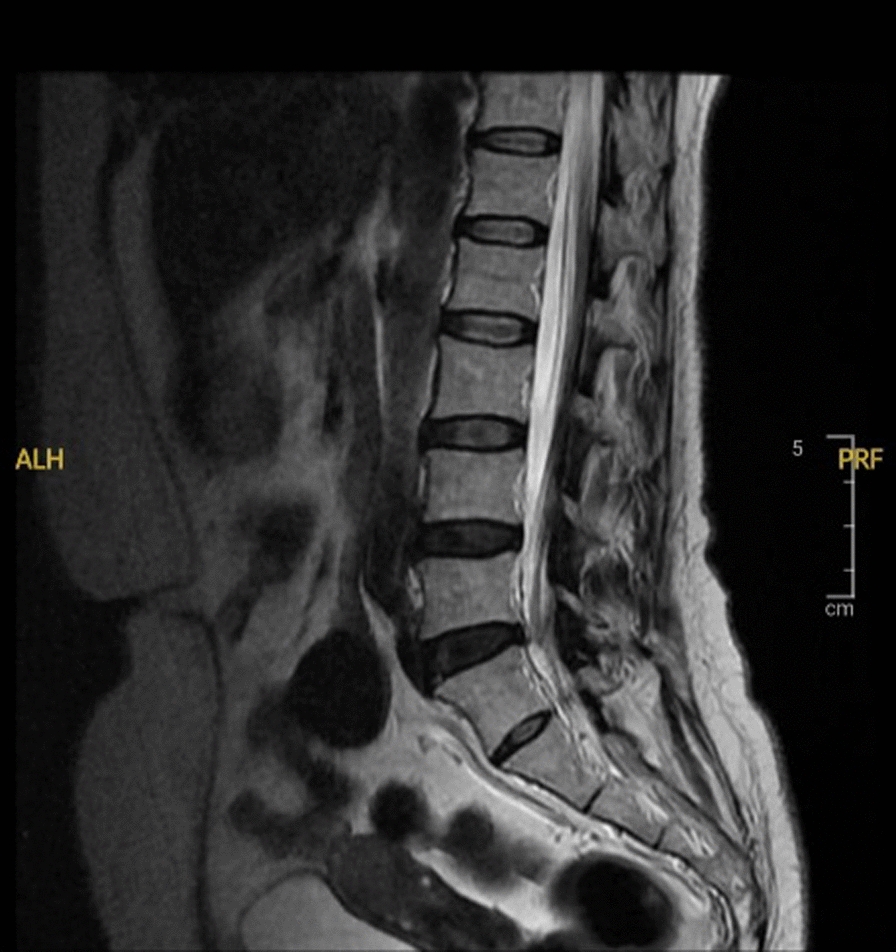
Lumbosacral magnetic resonance imaging of patient II
